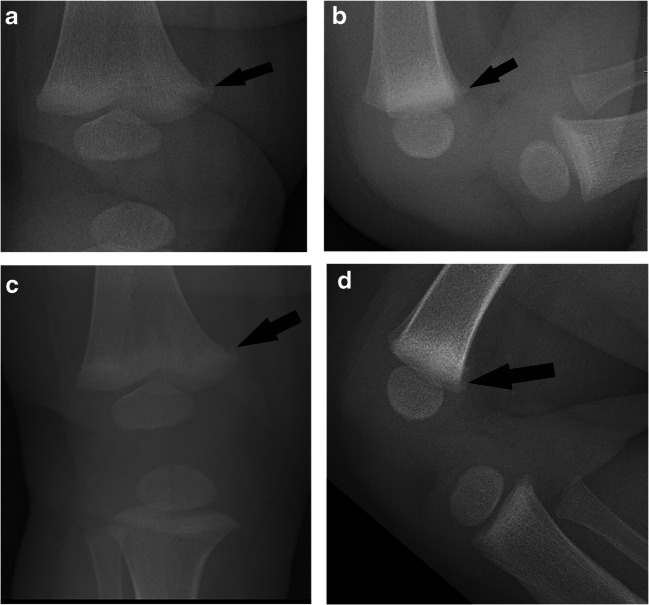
Distal right femur metaphyseal fragmentation in a 5-month-old boy. a Anteroposterior (AP) radiograph shows medial metaphyseal fragmentation (arrow). b Lateral radiograph shows posterior metaphyseal fragmentation (arrow). c Fourteen-day follow-up AP radiograph shows no interval change in the medial metaphyseal fragmentation (arrow). d Fourteen-day follow-up lateral radiograph shows no interval change in the posterior metaphyseal fragmentation (arrow). All radiologists reviewing separately the initial and follow-up radiographs blinded to clinical history diagnosed metaphyseal variation with moderate to high confidence on both the initial and follow-up radiographs. Seven radiologists diagnosed metaphyseal fragmentation both in the initial and follow-up radiographs, and one radiologist as metaphyseal step off
